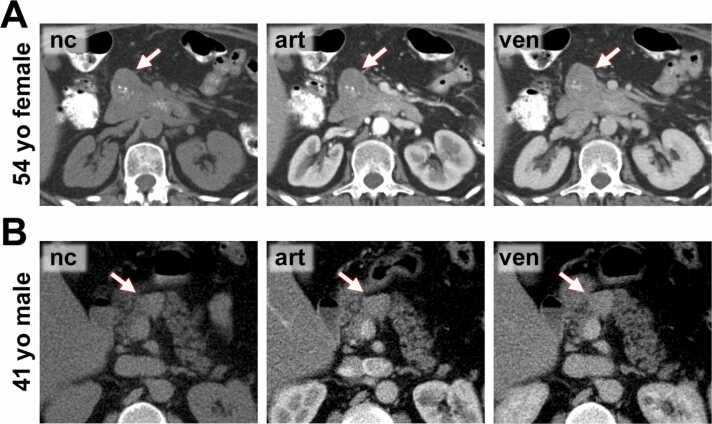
CT images from SPN patients. C) 54-year-old female patients with an SPN in the pancreatic head (*arrow*). The lesion is predominantly cystic with central calcifications (best seen in the non-contrast (nc) phase) and shows a capsule-like enhancement in the venous phase. D) 41-year-old male patient with a small SPN in the pancreatic body (*arrow*). The lesion is lesion is moderately hyperdense in the non-contrast, arterial (art), and venous phase. No capsule is apparent in CT. This lesion is very difficult to distinguish from pNEN.
